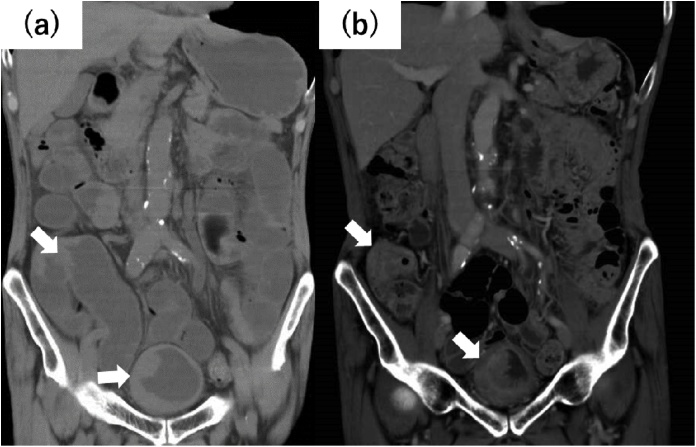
(a) Abdominal CT revealed construction findings of ileocecal and irregularity of the urinary bladder wall (white arrow). (b) Contrast-enhanced CT revealed a strong contrast effect (white arrow).
